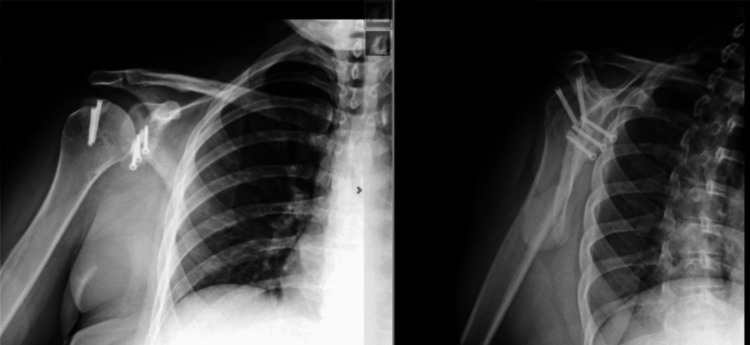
Anteroposterior (AP) and scapular Y radiographic views at 2 weeks after right shoulder iliac crest autograft glenoid fixation with proximal humerus osteochondral bone wedge allograft procedure.
